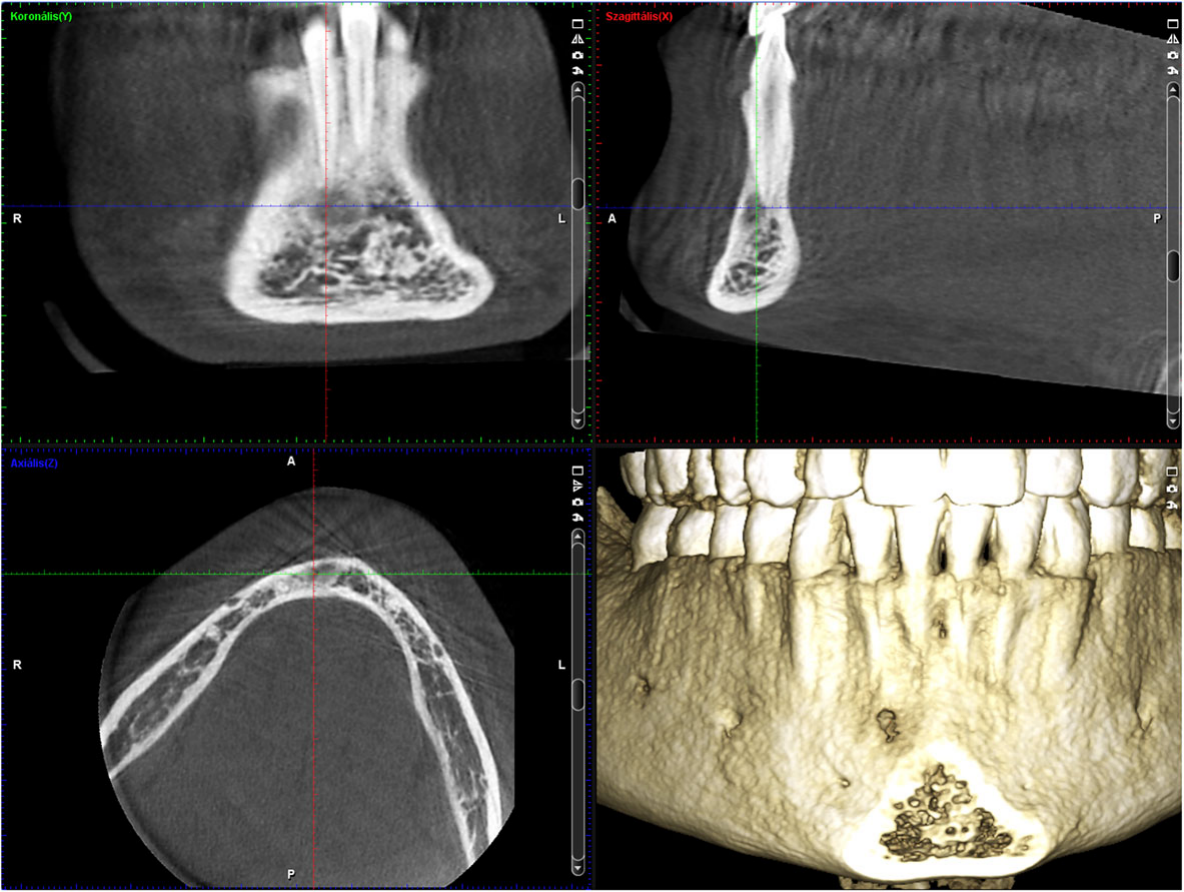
Early radiolucent lesion without radiopaque area were revealed around the root apex of 42 tooth on CBCT sections of patient 2. The inner surface of the buccal cortical begun to be eroded by the lesion. This is obvious when one compares this with the contralateral side
